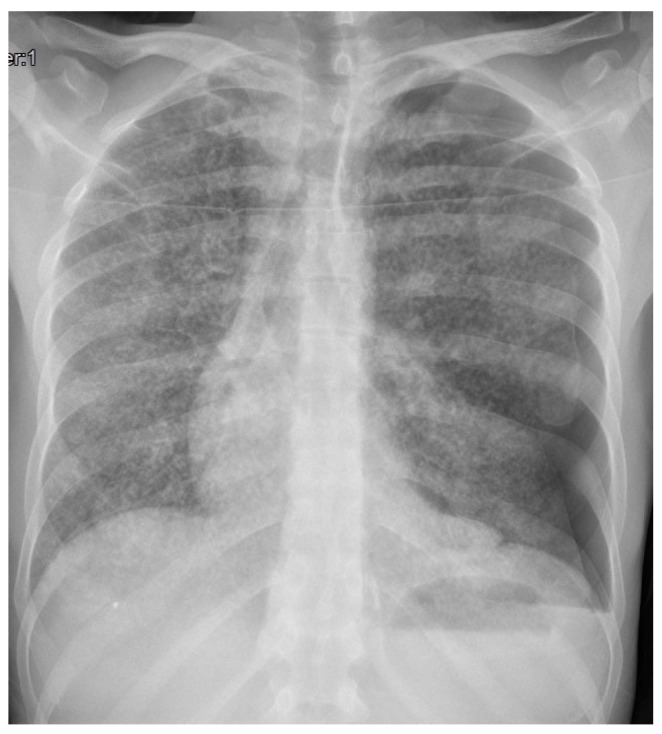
Chest X-ray of case n. 1.
